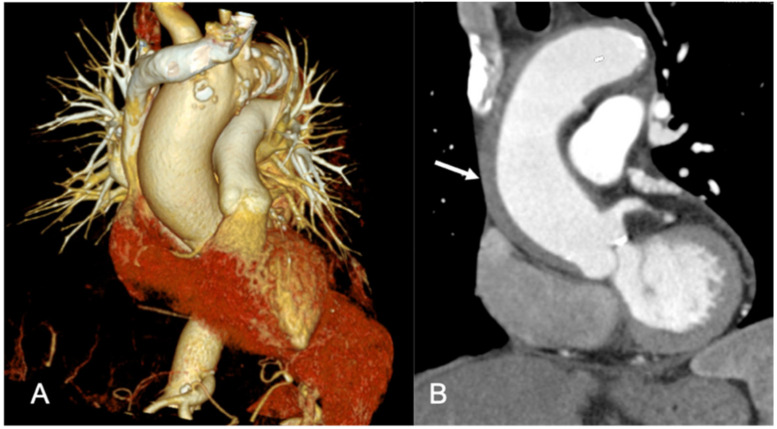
A 47-year-old woman with chest pain. (A,B) Combined CT evaluation of the aorta, pulmonary, and coronary arteries revealed type A dissection (arrow), no signs of pulmonary embolism or coronary stenosis.
